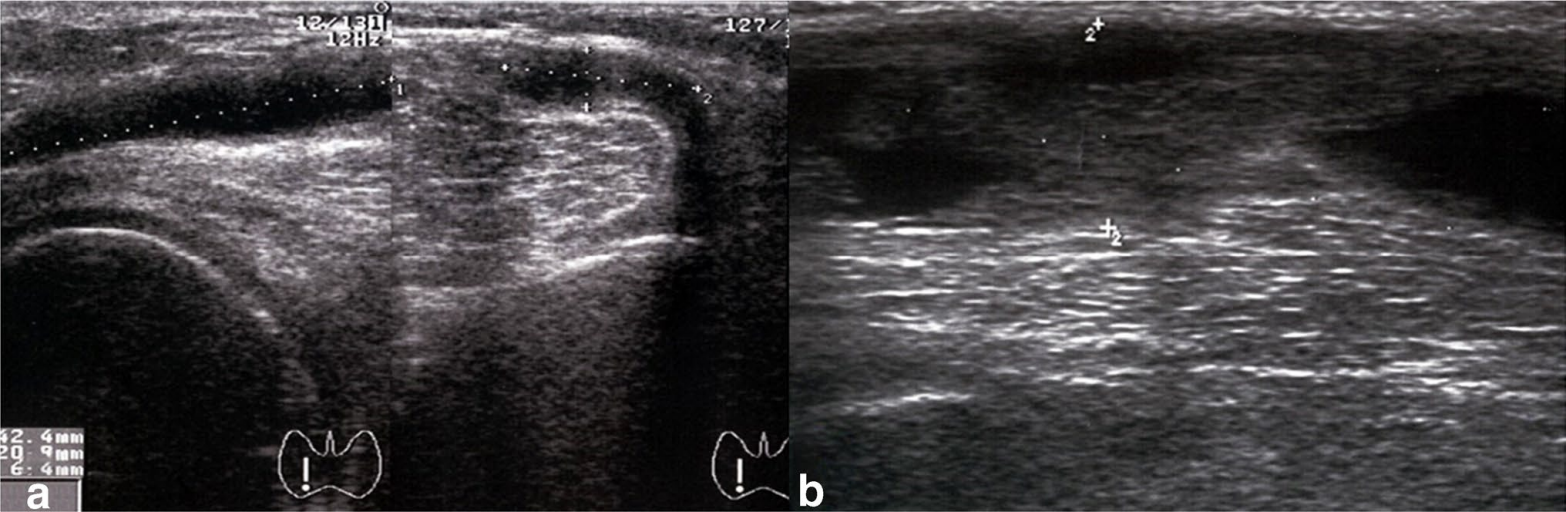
The recurrence of a popliteal cyst detected by means of the ultrasonography: (a) asymptomatic, slit-like cyst and (b) refilling and overgrowth of the cyst
